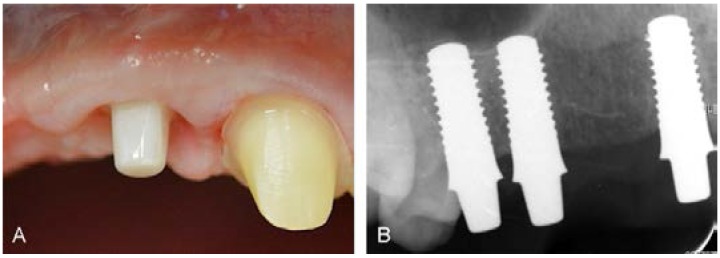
(A) Completely non-irritated peri-implant soft tissues of implant in region of 11 just prior to cementation of definitive prosthesis. (B) Radiographic investigation at nine months after implant placement, just prior to definitive cementation.
